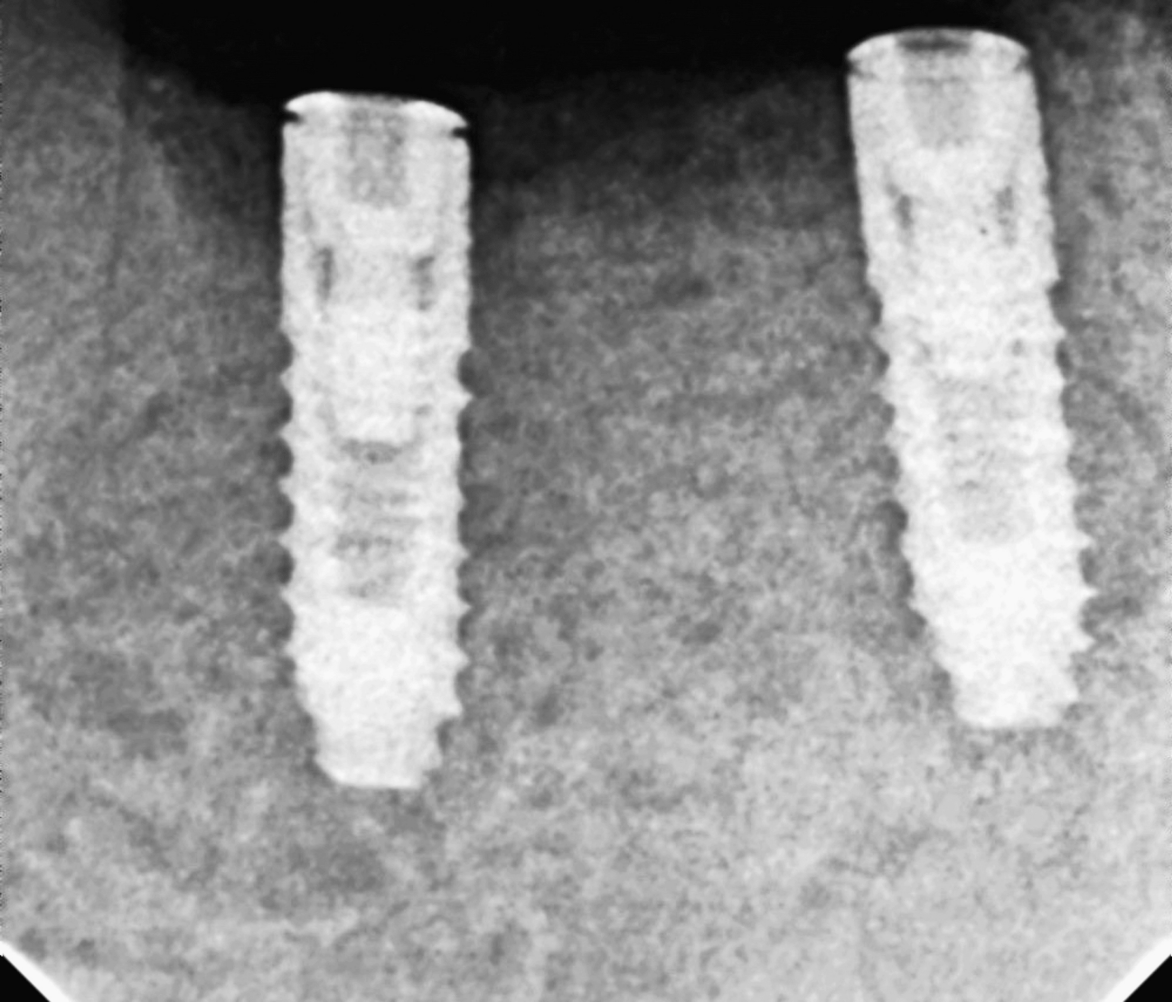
Radiograph after placement
